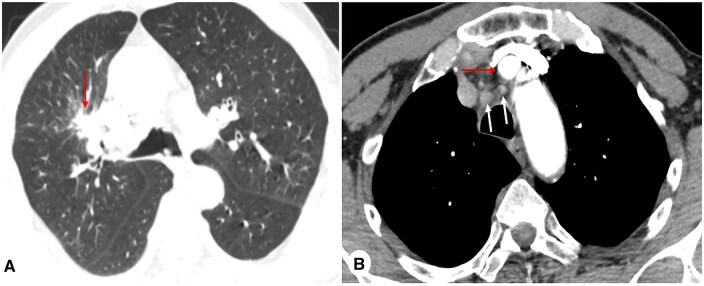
(A) CT scan showing the primary right upper lobe lesion (single arrow). (B) Mediastinal window showing the innominate artery (single arrow) and its close proximity to mediastinal lymph node station 2 R (two arrows).
